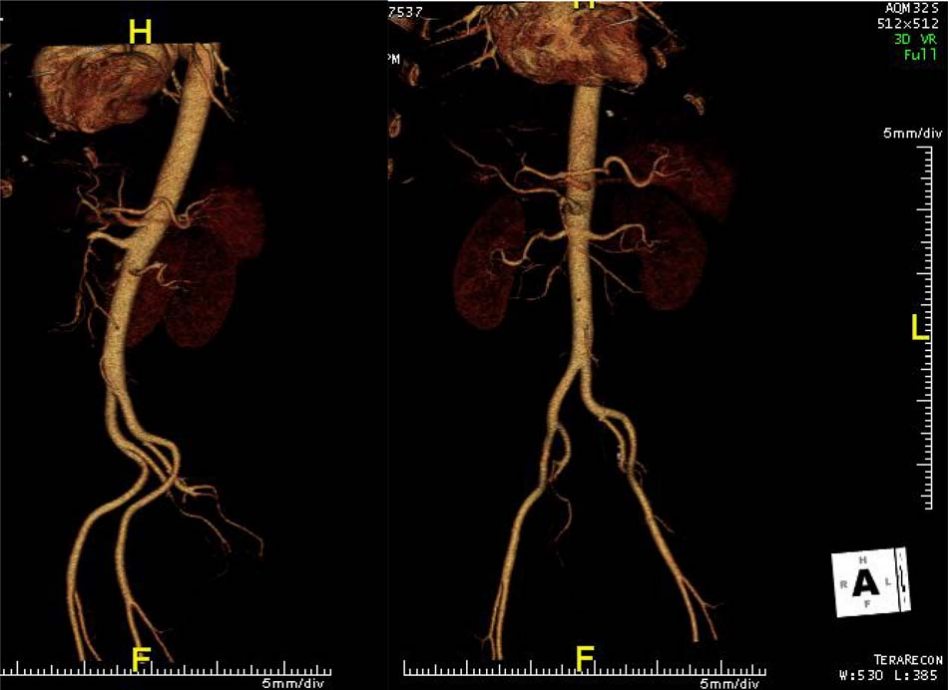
CT angiogram of abdominal aorta (sagittal plane on the left and coronal plane without bones on the right) demonstrating a narrow, hypodense SMA after the origin, raising the possibility of thrombus.
